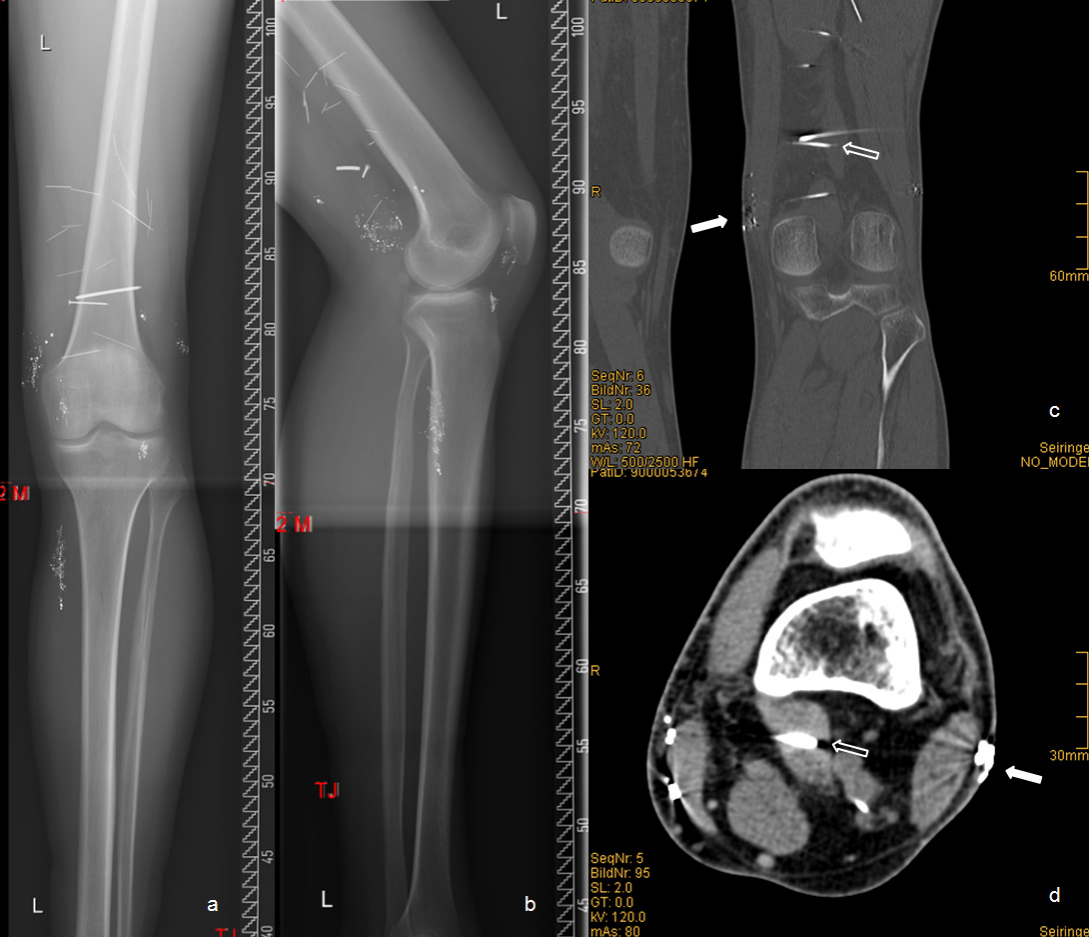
a-d. a&b) Preoperative anteroposterior and lateral radiographs of the left femur and tibia showing multiple radiopaque particles, spikes and spicules in the soft tissues around the knee. c&d) Coronal and transversal CT scan of the left knee and the affected soft tissues showing several metallic foreign bodies (hollow arrow: 2 spikes) and mercury depots (white arrow).
